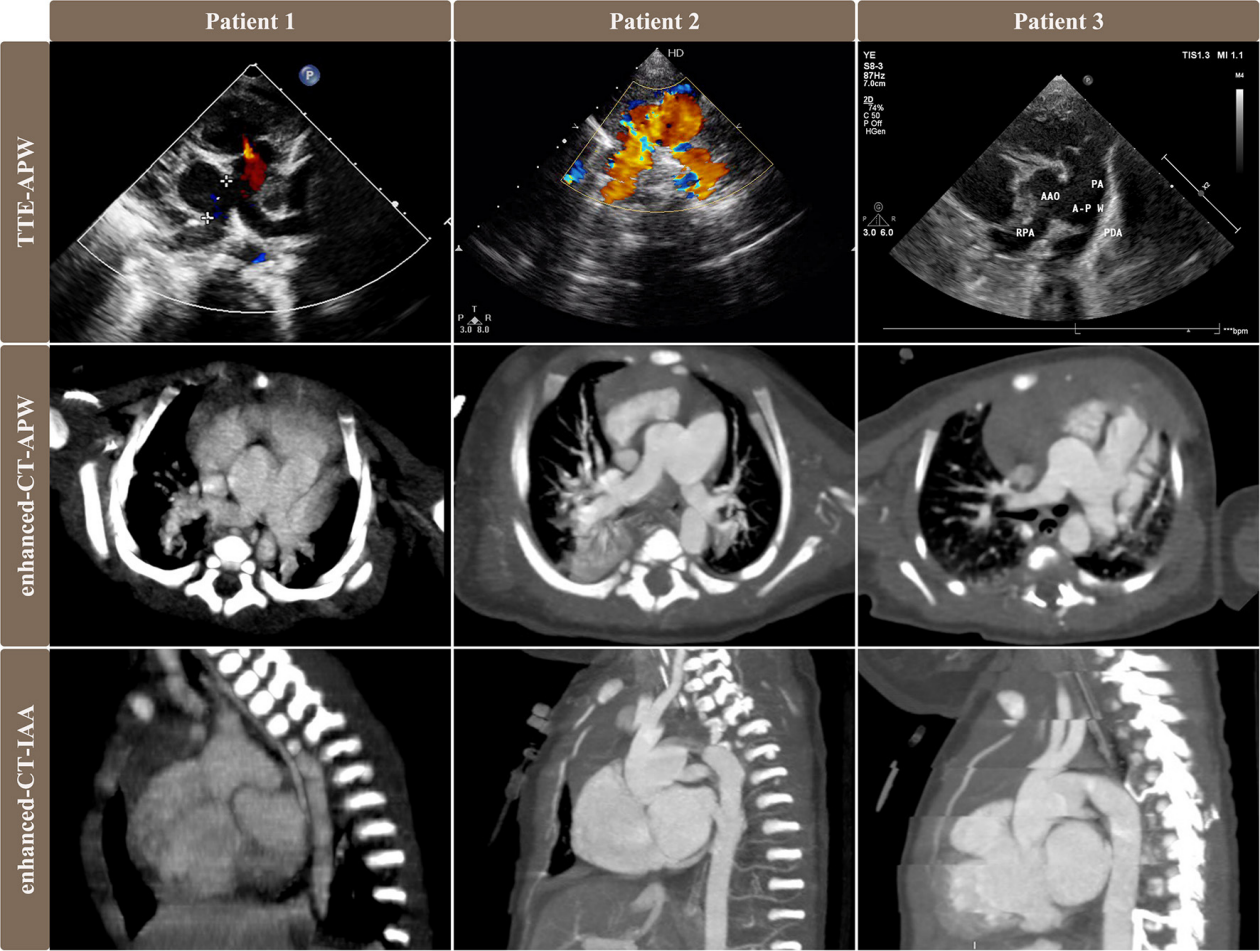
Pre-operative images of transthoracic echocardiography and contrast-enhanced CT scan showed different types of APW, AORPA, and IAA in 3 patients. Images of the first and second rows showed the relationship among RPA, AAO, APW, and PA. Patient No. 1 was APW type IIa, patient No. 2 was APW type III, and patient No. 3 was APW type IIb. Images of the third rows were sagittal views of CT exhibiting IAA that descending aorta originated from PDA.TTE, transthoracic echocardiography; APW, aortopulmonary window; AORPA, the aortic origin of the right pulmonary artery; IAA, interrupted aortic arch; RPA, right pulmonary artery; AAO, ascending aorta; PA, pulmonary artery; PDA, patent ductus arteriosus.
